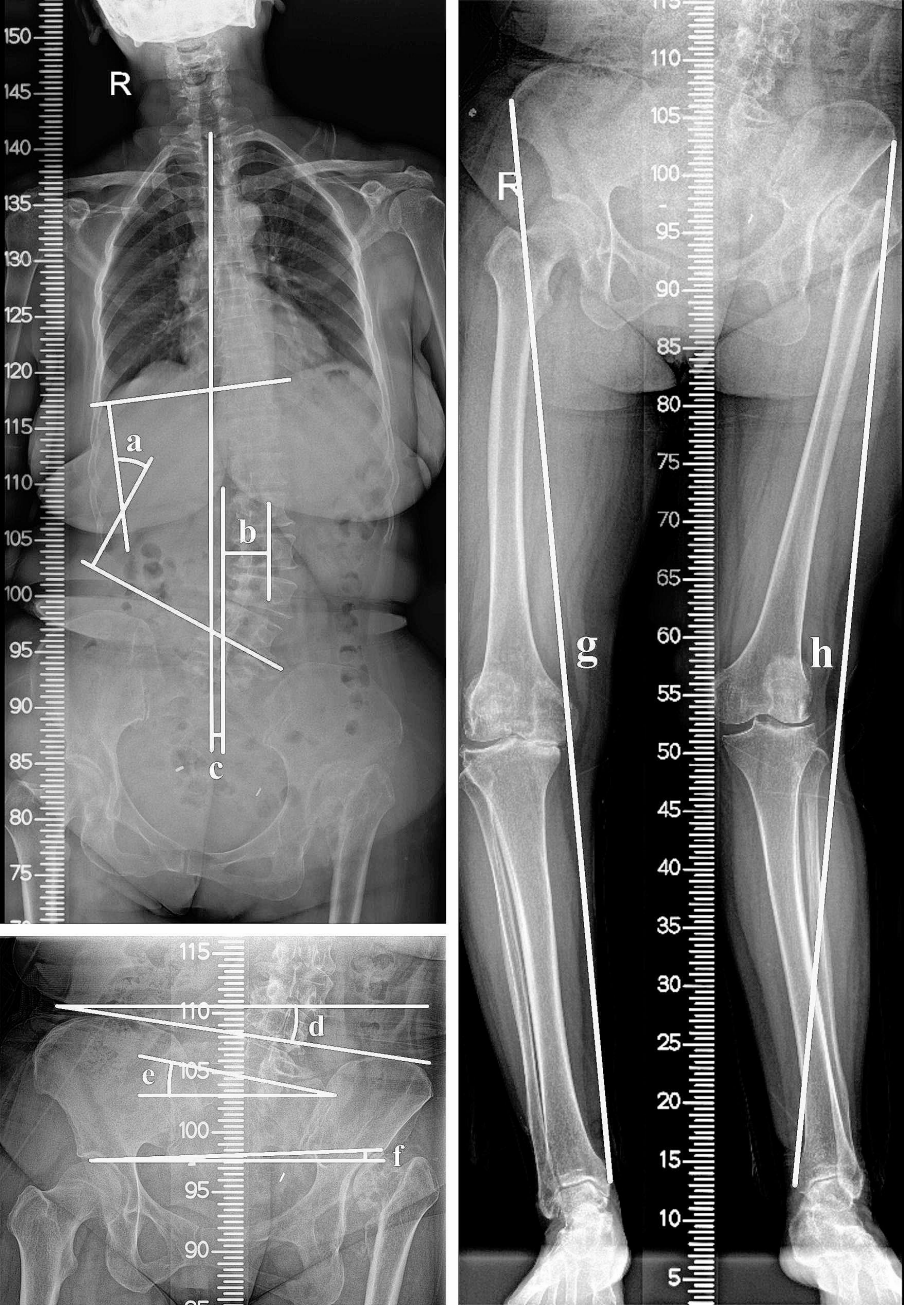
Measurements of radiographic evaluations. (a) Cobb angle; (b) Apical vertebral translation (AVT); (c) Coronal decompensation (CD); (d) Sacral obliquity (SO); (e) Iliac obliquity (IO); (f) Hip obliquity (HO); (g) Healthy lower limb length (HLLL); (h) Affected lower limb length (ALLL)
